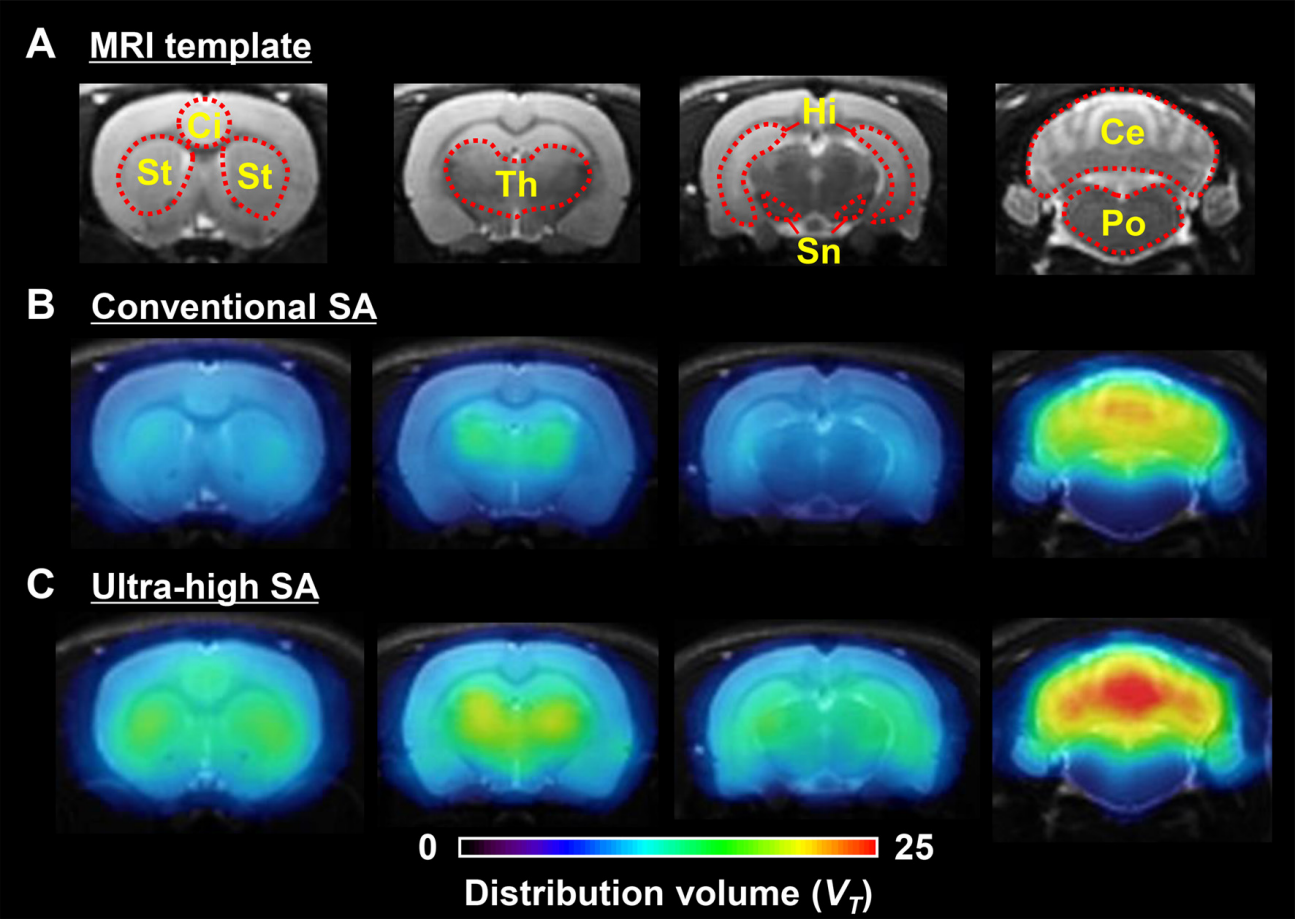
Representative parametric PET/MRI fused images based on *V*
